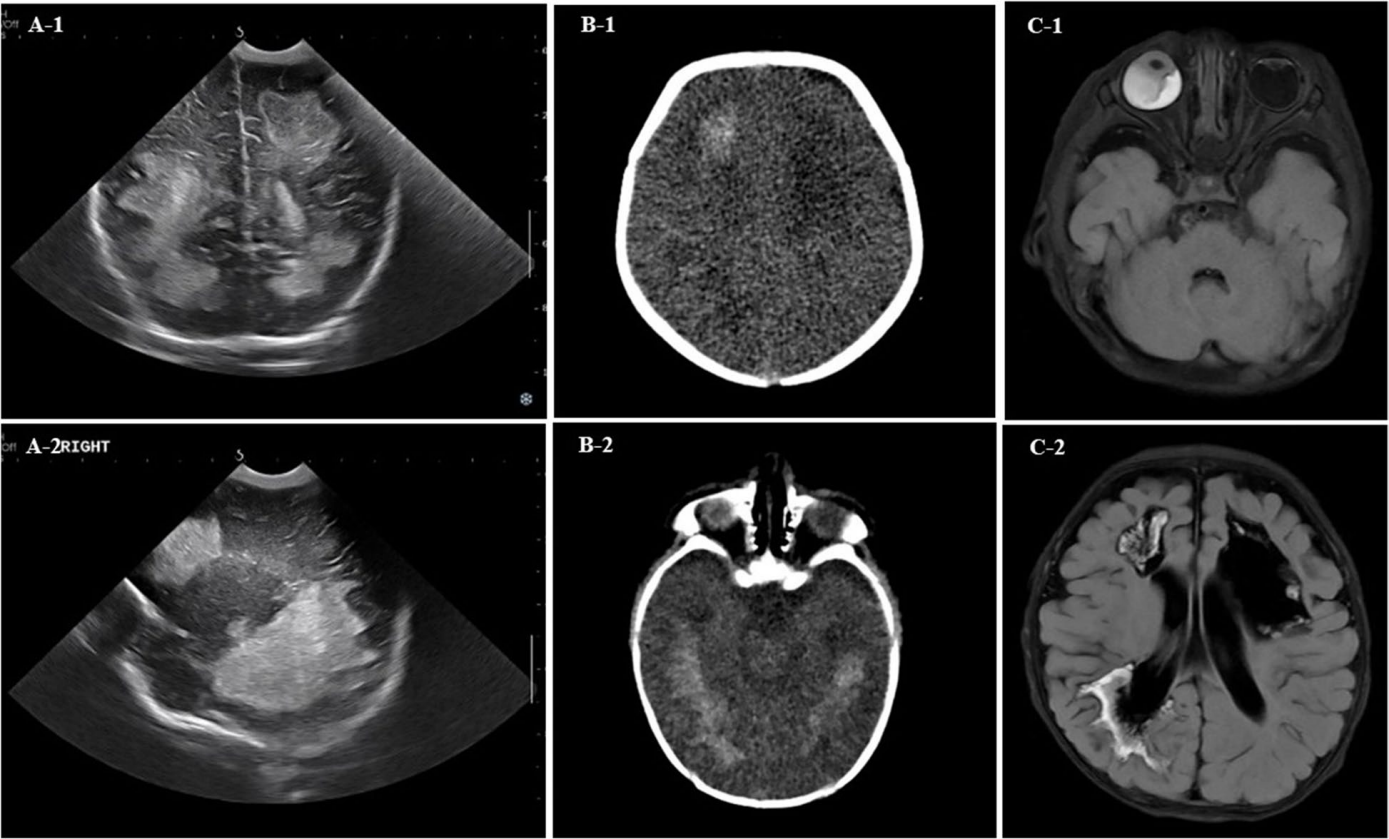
Brain images of the patient. (A) Brain sonography of neonate on postnatal day 6 showing recent onset hemorrhagic infarction and liquefied infarction from possible in-utero insult. (B) Brain computer tomography (CT) images of neonate on postnatal day 6 showing intracranial hemorrhage, old ischemic lesions, and suspicious intraventricular hemorrhage. (C) Brain MRI (T2 FLAIR image) obtained at 1 month of age, showing cystic cerebromalatic changes in the bilateral periventricular white matter, diffusion restrictions along the margin of the previously infarcted area, suggesting ongoing loss of ischemic tissue, and right retinal detachment with vitreous hemorrhage
